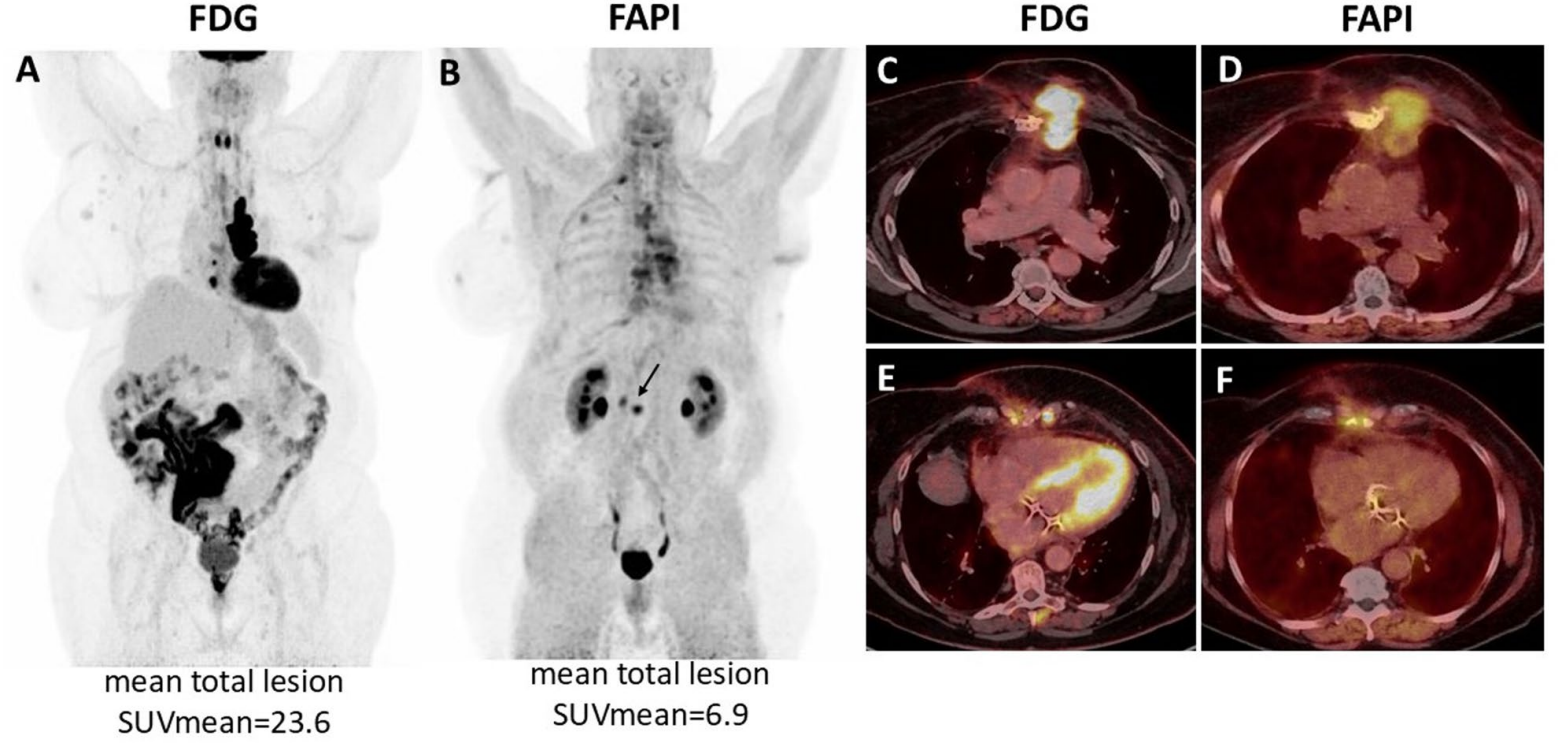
Superior detection performance of [^18^F]FDG PET/CT, compared to [^68^Ga]Ga-FAPI-46 PET/CT. 65 years old woman diagnosed with left-sided ductal luminal B-like breast cancer (UICC stage 3c). PET/CT showed abnormal uptake in a soft tissue mass involving the left para-sternal region of the thoracic wall (SUV_max_ [^18^F]FDG 42.3 vs. [^68^Ga]Ga-FAPI-46 7.6 - C&D), internal mammary lymph nodes (SUV_max_ [^18^F]FDG 18.9 vs. no [^68^Ga]Ga-FAPI-46 uptake - E&F), and bone (SUV_max_ [^18^F]FDG 4.1 vs. [^68^Ga]Ga-FAPI-46 9.8 (arrow in B)). Overall lesions presented higher [^18^F]FDG uptake than [^68^Ga]Ga-FAPI-46 uptake (mean total lesion SUV_mean_ on [^18^F]FDG 23.6 vs. [^68^Ga]Ga-FAPI-46 6.9). A&B - PET maximum intensity projection; C-F - PET/CT fused transaxial plans
